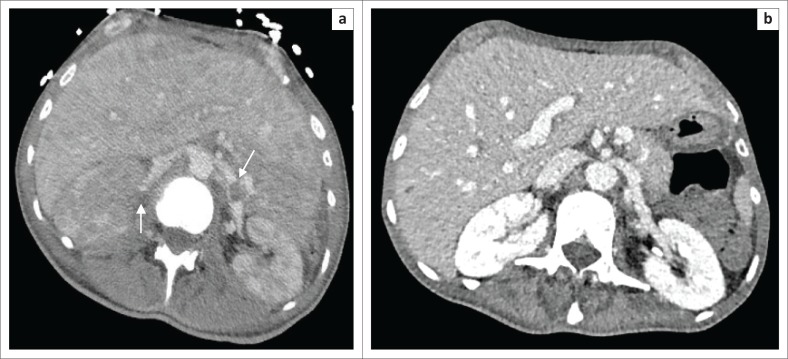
(a) Axial post-contrast abdominal CT demonstrates bilateral renal vein tumour thrombi (arrows) with extensive renal tumour infiltration, most marked on the right. The tumour thrombi have resolved on (b) post-contrast imaging obtained 1 month later, and there has been a marked reduction in renal tumour volume.
